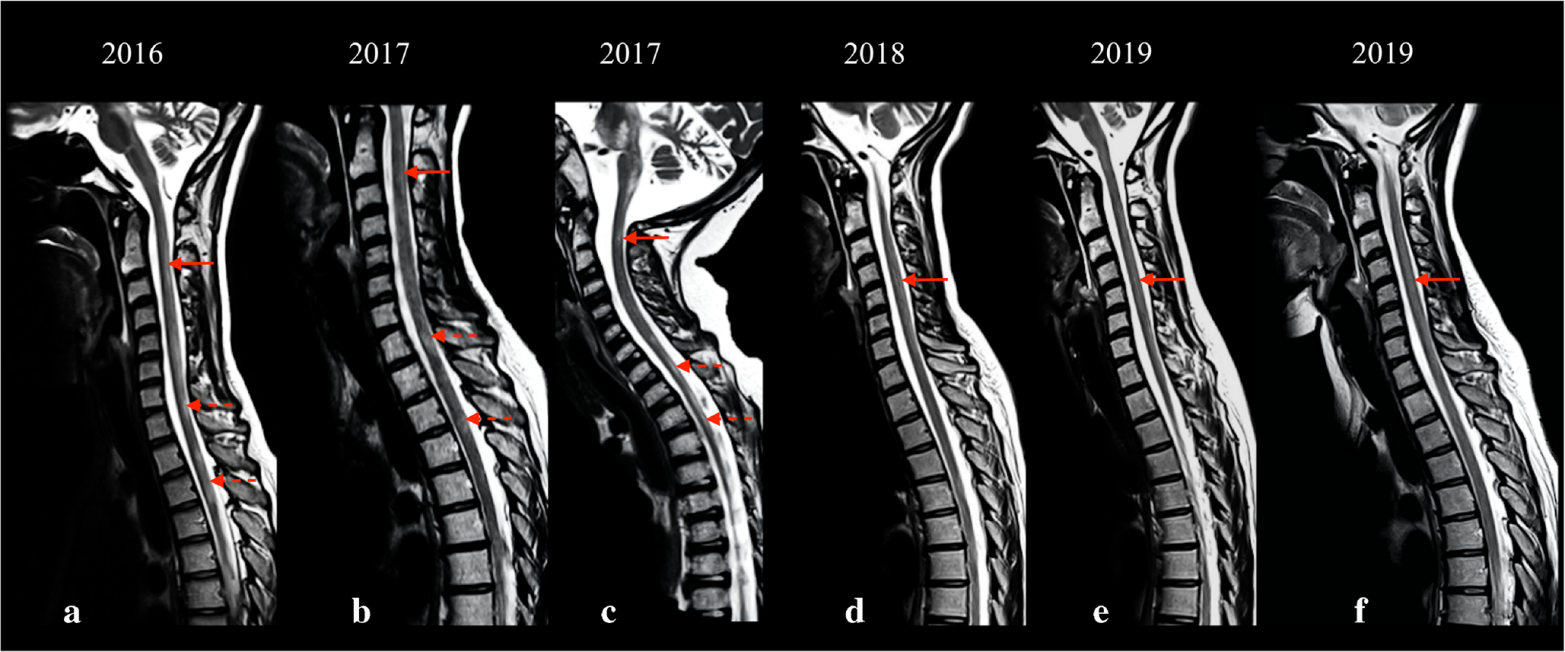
Sagittal T2-weighted images displaying spinal cord involvement across 4 years in a patient who had sudden onset of ambulation loss, with widespread “patchy” lesions in the cervical (images a, b, solid arrow) and dorsal (images a, b, dotted arrows) segments of the spinal cord. The lesions showed partial remission with more focal appearance (image c), and gradual worsening over the follow-up, with diffuse spinal cord hyperintensity (d–f, solid arrows)
